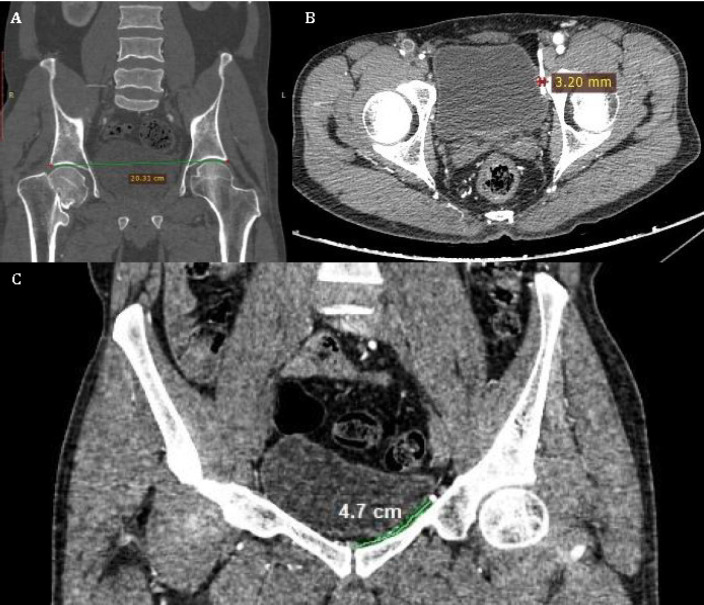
2D coronal reconstruction of CT angiographic image in a 40-year-old woman showing the measurement of the inter-acetabular distance between the right and the left acetabulum upper labarum (A); 2D axial section of CT angiographic image in a 35-year-old man showing the measurement of diameter of the vessel (left side in this case) (B); 2D coronal reconstruction of CT angiographic image (soft tissue window) in a 42-year-old woman. The distance between the symphysis and the Corona Mortis (left side in this case) is measured by using the free hand line (C).
